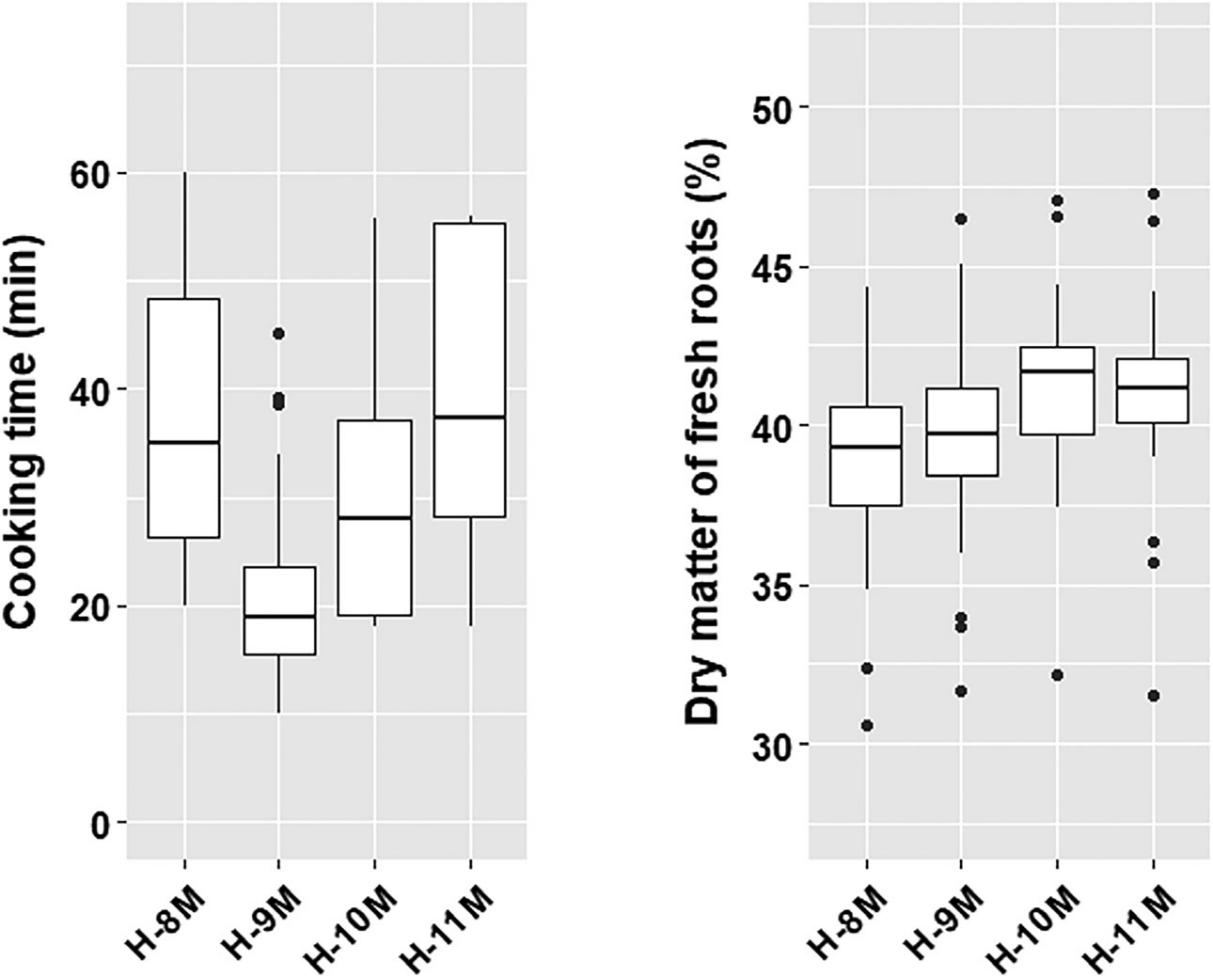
The effect of harvest time on cooking time (CT) and dry matter content (DMC). Significant difference (*P* < 0.05) in CT was observed among harvest time, but not in DMC.
